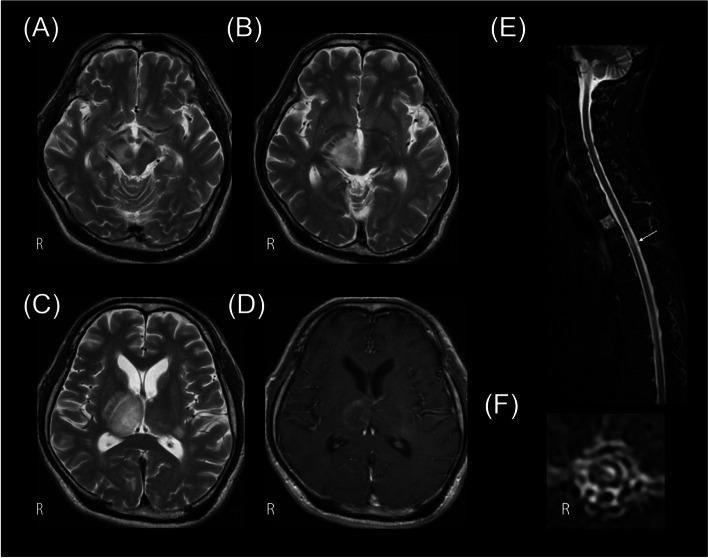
Transaxial brain magnetic resonance imaging (MRI) 1 month after neurological onset, showing T2 high intensity lesion in (A) the right cerebral peduncle, (B) diencephalon to (C) thalamus, adjacent to the third ventricle with (D) gadolinium enhancement. Spinal cord MRI 2 months after neurological onset showing short inversion recovery (STIR) images on (E) sagittal and (F) transaxial plane with bright spotty lesion at T3 level
